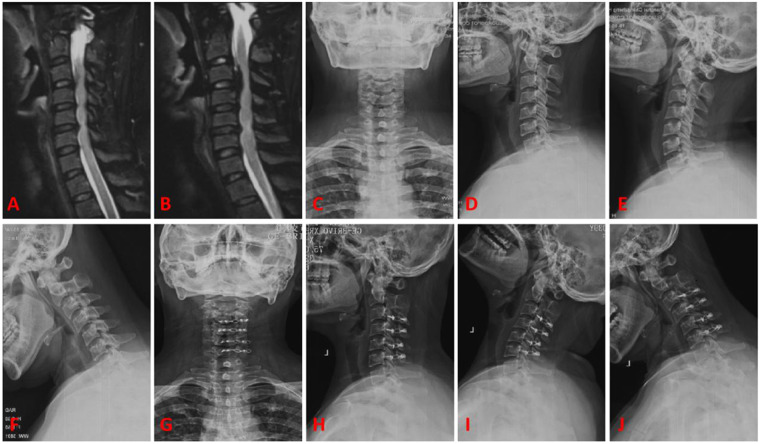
Typical case 2. (A,B) Preoperative sagittal MRI images, showing varying degrees of stenosis at the C3/4, C4/5, and C5/6 levels, with ossification of the posterior longitudinal ligament at C3/4. (C,D) Preoperative anterior-posterior x-rays, showing slight straightening of the cervical curvature. (E,F) Preoperative flexion-extension x-rays, showing instability of the C2 vertebra. (G,H) Postoperative follow-up lateral x-rays, showing good position of the internal fixation. (I,J) Postoperative follow-up flexion-extension x-rays, showing no significant changes in the instability of the C2 vertebra.
